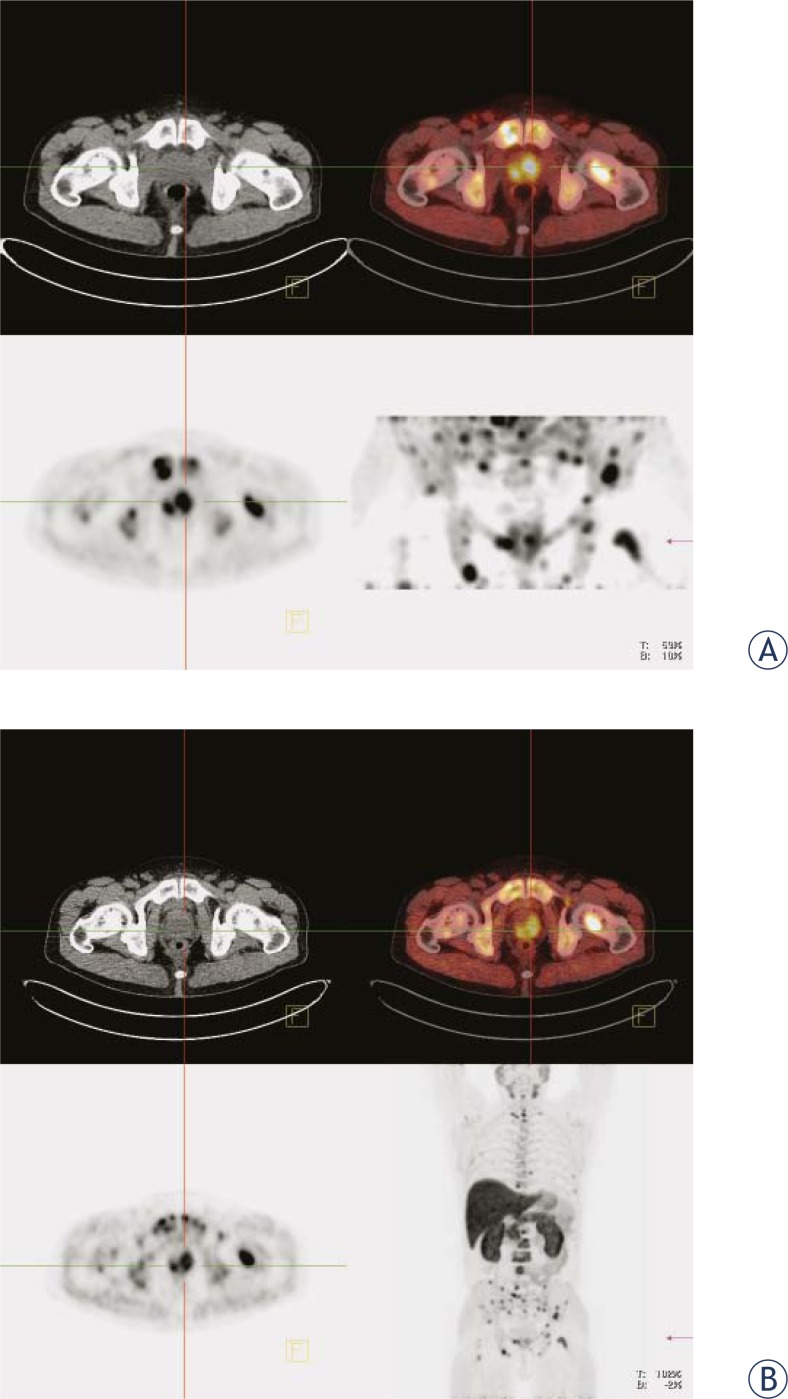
Bone metastases due to prostate cancer: A prostatic bed (early images), B whole body (late images). Upper left panel: CT image. Upper right panel: fused PET/CT image. Lower left panel: PET image. Lower right panel: maximum intensity projection (MIP).
